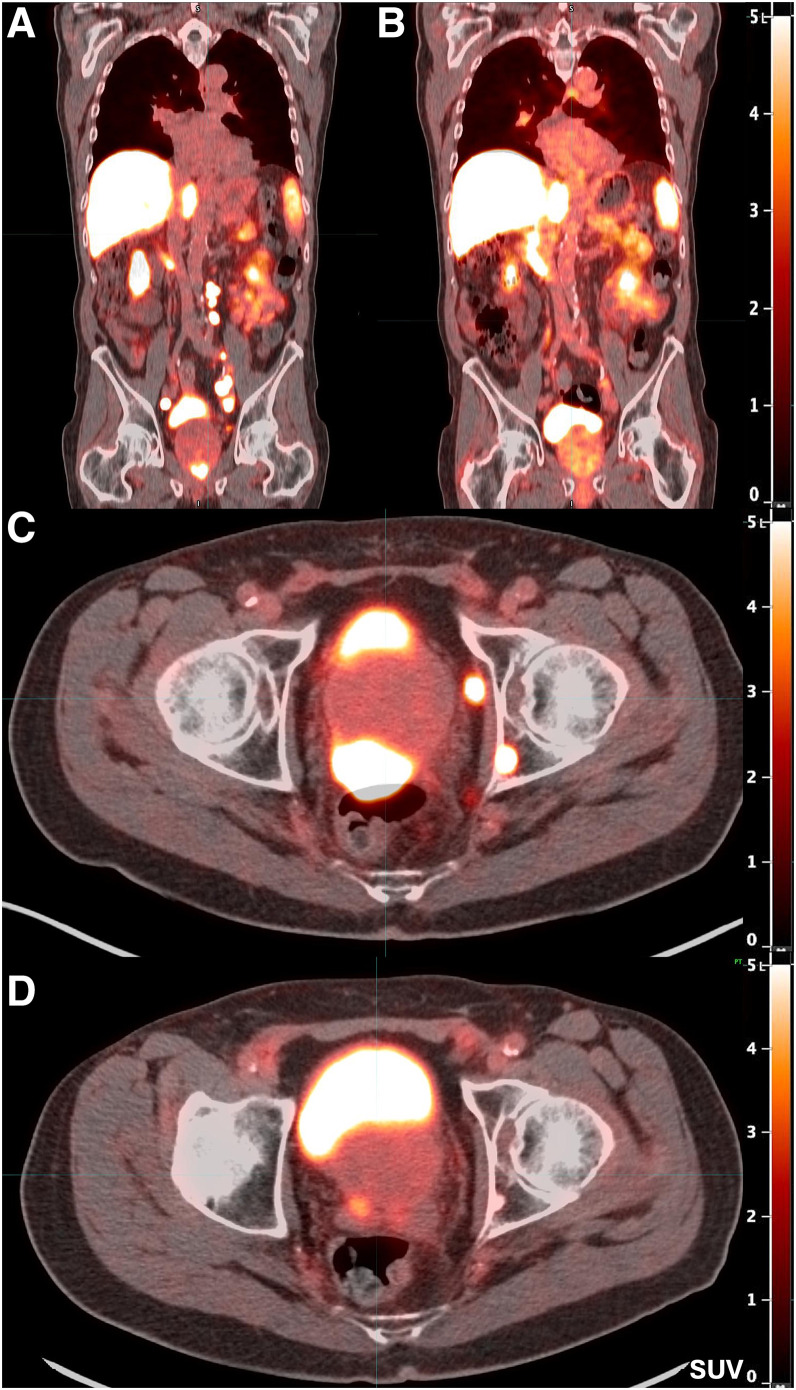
Burden of PSMA-expressing prostate cancer before and after treatment with 2 cycles of [^177^Lu]Lu-PSMA-617. (A and B) Reduction in overall disease burden is evident after treatment. Avidity of patient’s primary prostate tumor and his osseous metastasis shown before (C) and after (D) treatment.
